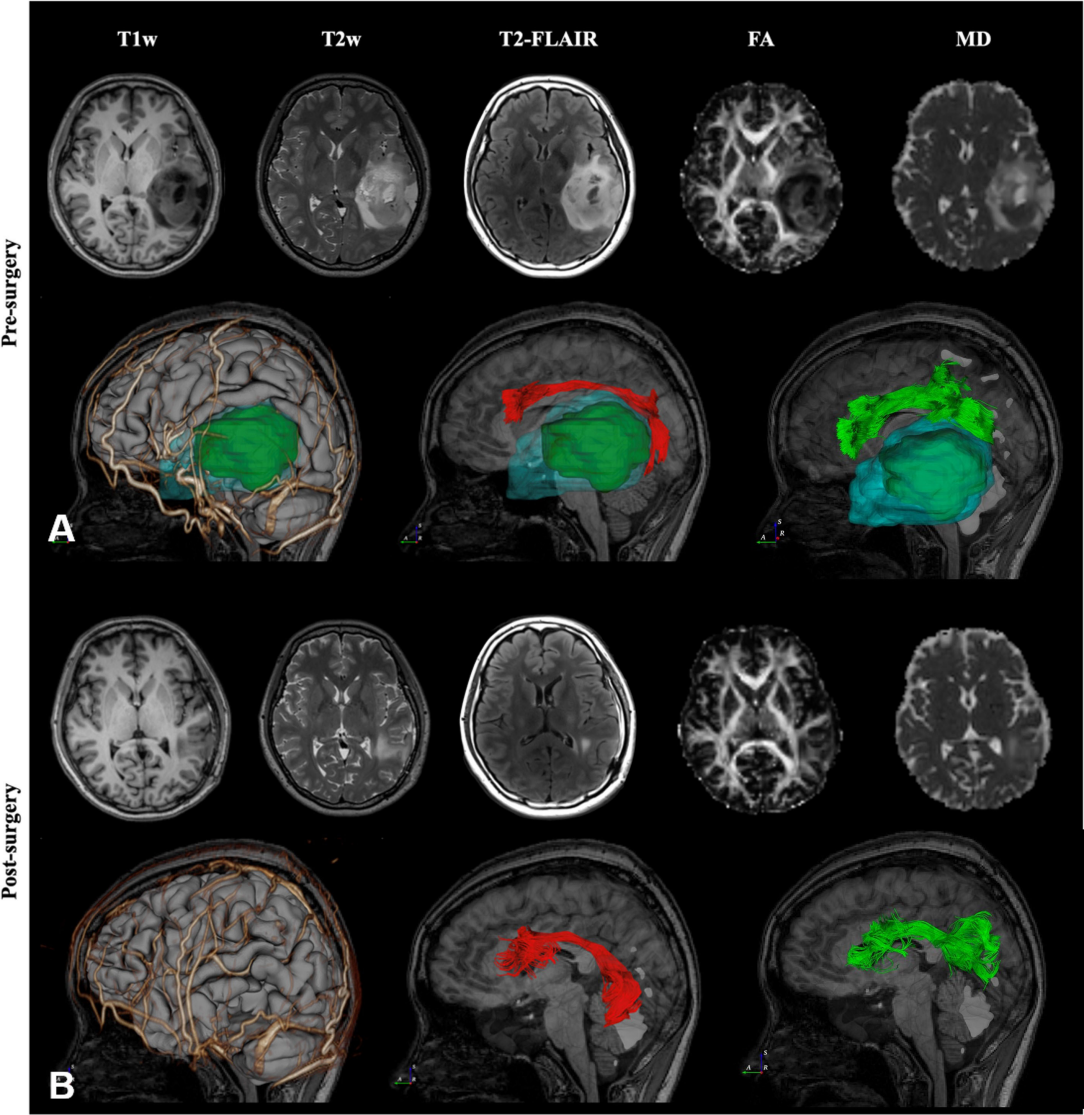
Preoperative and post-operative imaging of a patient with left temporal lobe astrocytoma. Case 2, A 41-year-old woman with temporal lobe glioblastoma over the left temporal lobe with mild perifocal edema was reconstructed and displayed in DiffusionGo (A). The AF (red) and SLF-II (green) were automatically reconstructed and integrated with the cortical surface (gray), arteries and veins (gold), tumor (dark green), and peritumoral edema (light blue with translucent). Superior displacement of the left Wernicke’s area was identified with intact AF projecting to the left premotor and left Broca’s areas. After a complete survey, since the patient could not endure an awake surgery, tumor removal was performed under general anesthesia without any neurological deficits postoperatively. Two months later, MRI of the brain revealed total gross removal without residual tumor, and DTI showed preservation of the AF (red) and SLF-II (green) (B). (FA, Fractional Anisotropy).
